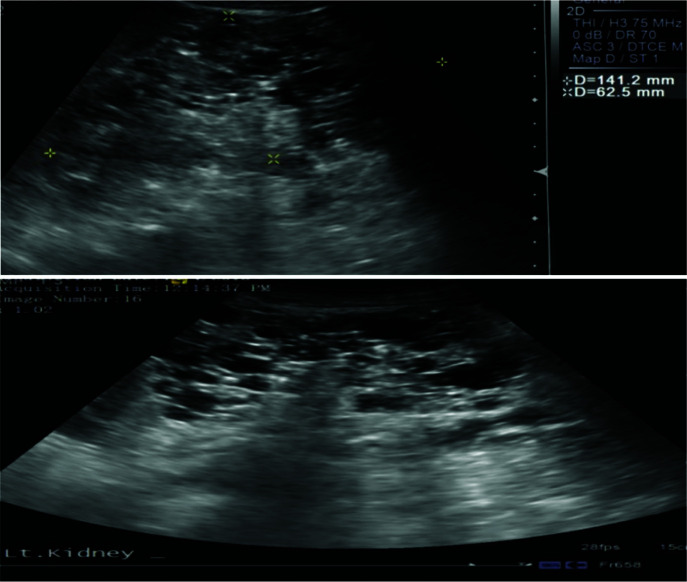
Ultrasound imaging reveals that both kidneys are enlarged with multiple cysts and loss of normal architecture, suggestive of polycystic kidney disease.
